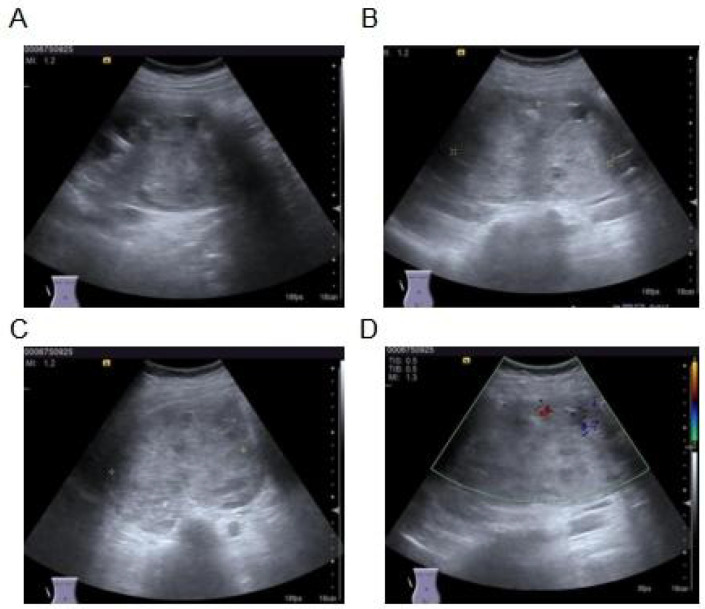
Ultrasound analysis images. (A) Longitudinal view: A large isoechoic mass (measuring approximately 120 × 104 × 75 mm) centered in the renal pelvis region, distorting the normal renal architecture. (B) Long-axis view: Uneven internal echo distribution with scattered small cystic necrotic areas within the mass. (C) Short-axis/transverse view: The mass has relatively clear boundaries and causes marked compression and thinning of the adjacent renal parenchyma. (D) Color Doppler flow imaging (CDFI): Minimal to no detectable internal vascularity within the mass.
